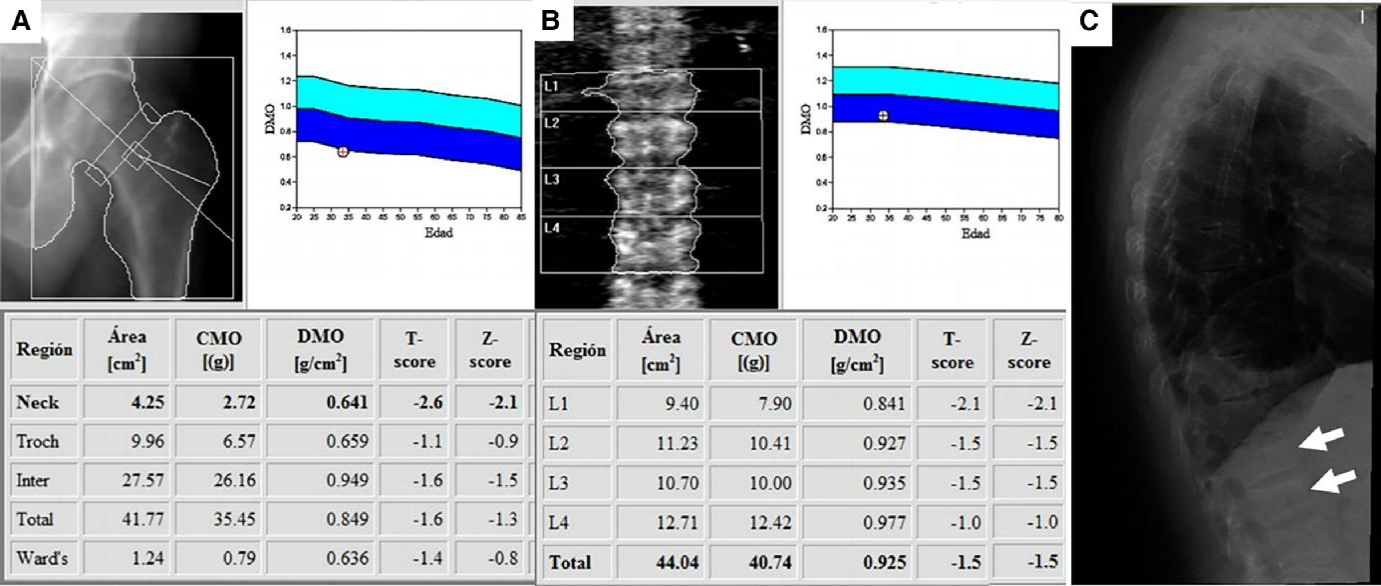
(A) Dual-energy X-ray absorptiometry (DXA) scan of the femoral neck and total hip showing low bone mineral density. (B) DXA scan of the lumbar spine showing low bone mineral density. (C) Lateral X-ray of the lumbar spine showing diminished vertebral body height with wedge deformity, indicative of vertebral fractures (white arrows).
